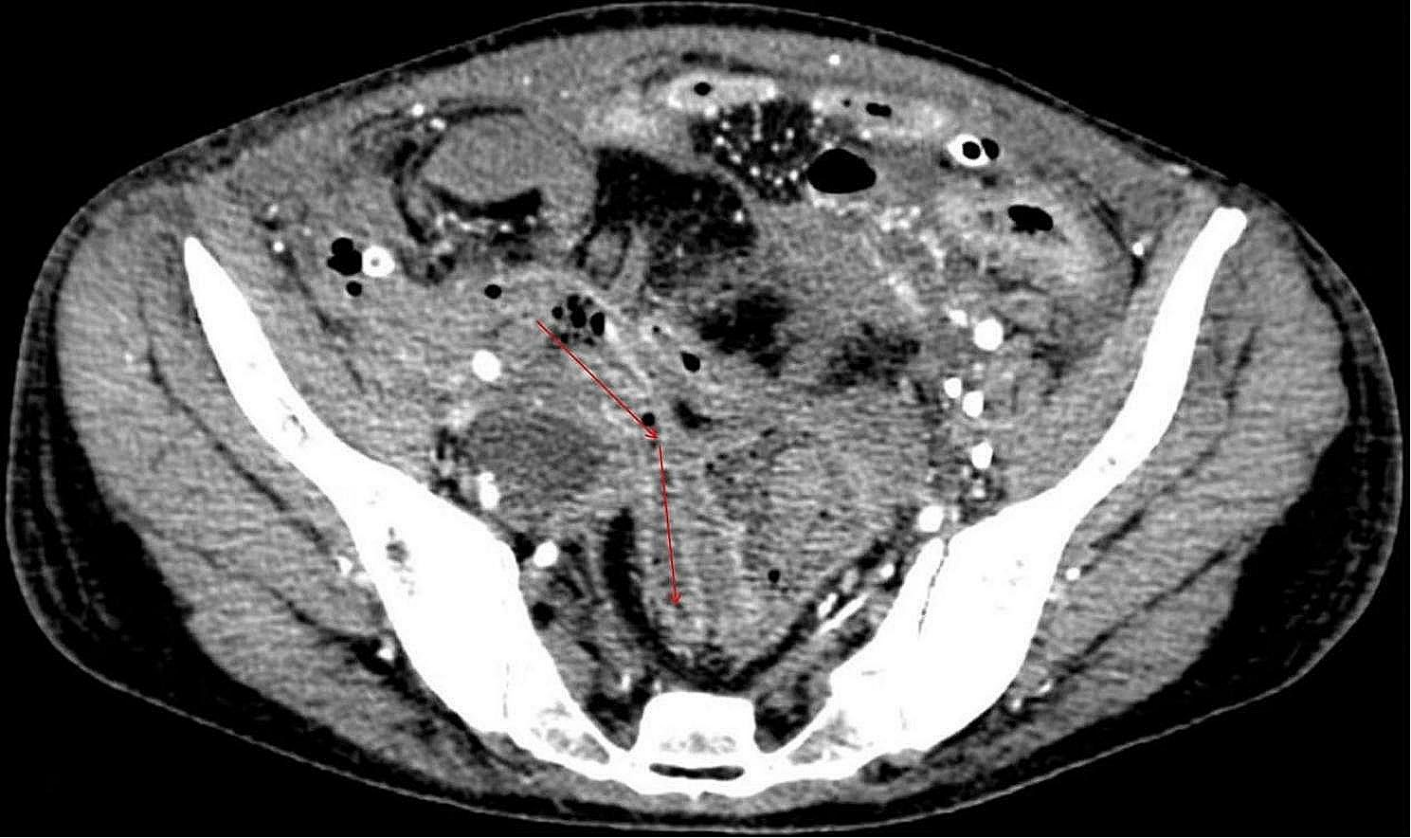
Computed tomography image showing an intestinal fistula (red arrow)
